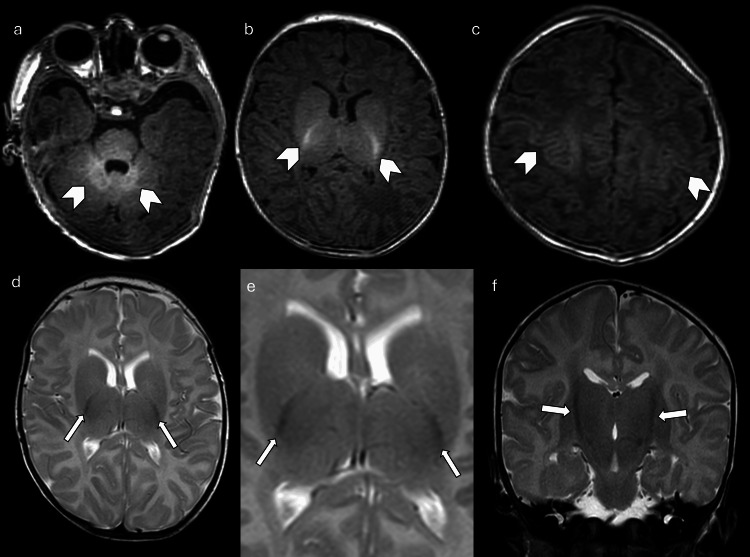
Normal myelination at birth (a), (b), and (c): Axial T1W images showing T1 shortening in the dorsal brainstem, posterior limb of internal capsule, lateral thalamus, central centrum semiovale, and perirolandic region (arrowheads) in term neonates serve as essential references to identify pathological deviations. (d), (e), and (f): Axial and coronal T2 images demonstrate T2 hypointensity in the posterior limb of the internal capsule (arrows) that corresponds to areas of normal early myelination. Images obtained by the authors from the institutional PACS. All images are de-identified and used in accordance with institutional policy. T1W: T1-weighted; PACS: picture archiving and communication system
